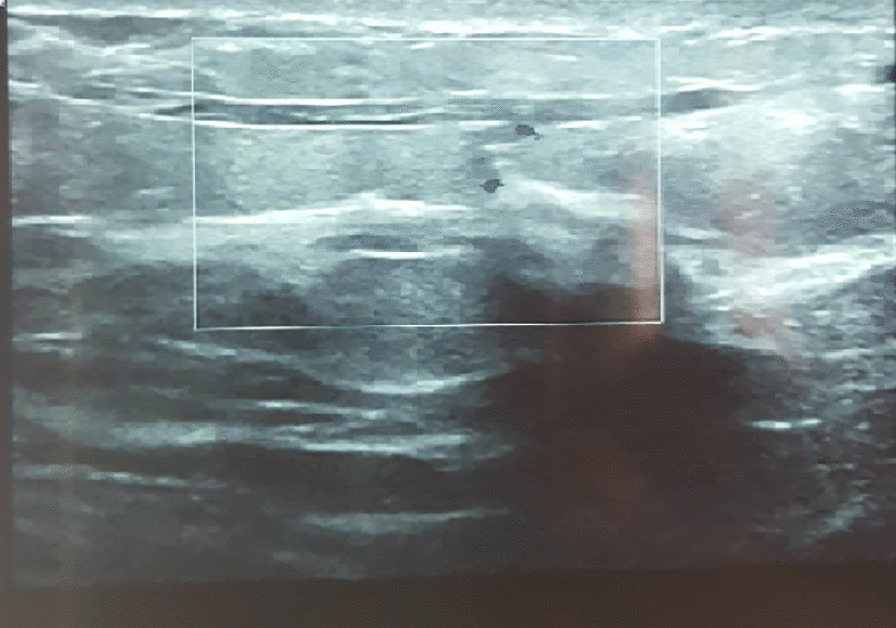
Intraluminal thrombus on sonography
